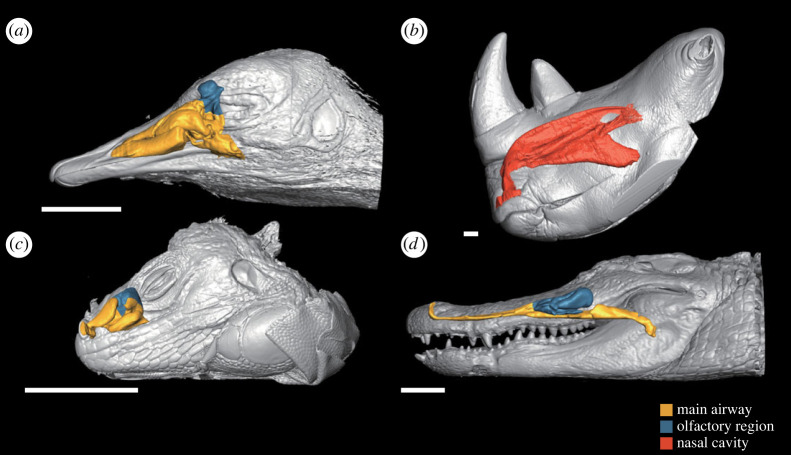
Three-dimensional reconstructions of the nasal cavities of extant endotherms (*a*,*b*) and ectotherms (*c*,*d*) based on CT scan data. (*a*) *Struthio camelus* (Aves; OUVC 10491), (*b*) *Ceratotherium simum* (Mammalia; OUVC 9754), (*c*) *Iguana iguana* (Lepidosauria) and (*d*) *Alligator mississippiensis* (Crocodylia; OUVC 9761). Scale bars equal to 5 cm.
